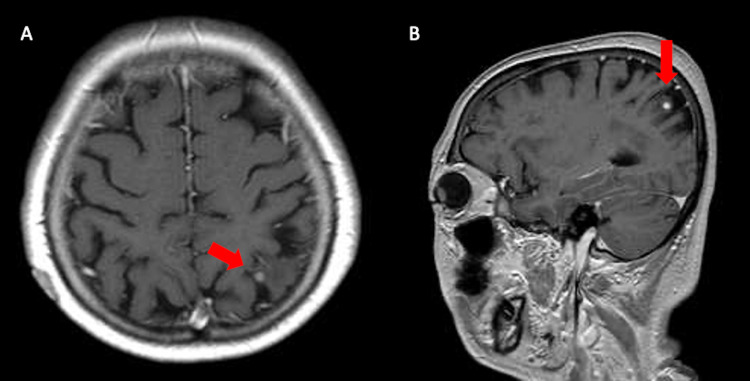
MRI of the head two years after the recurrence. These are (A) horizontal and (B) sagittal sections of a gadolinium contrast-enhanced T1-weighted MRI performed for screening. A 4 mm strongly contrasted nodule (red arrow) was seen in the left parietal lobe with surrounding edema. There were no other lesions suspicious for metastasis.
